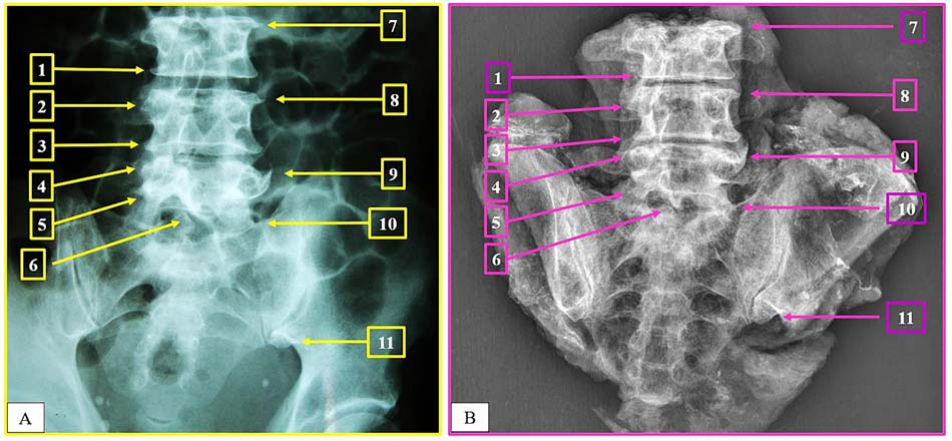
Intravitam (A) and postmortem (B) X-ray confrontation of the lumbar spine. Matching points 1–11.
